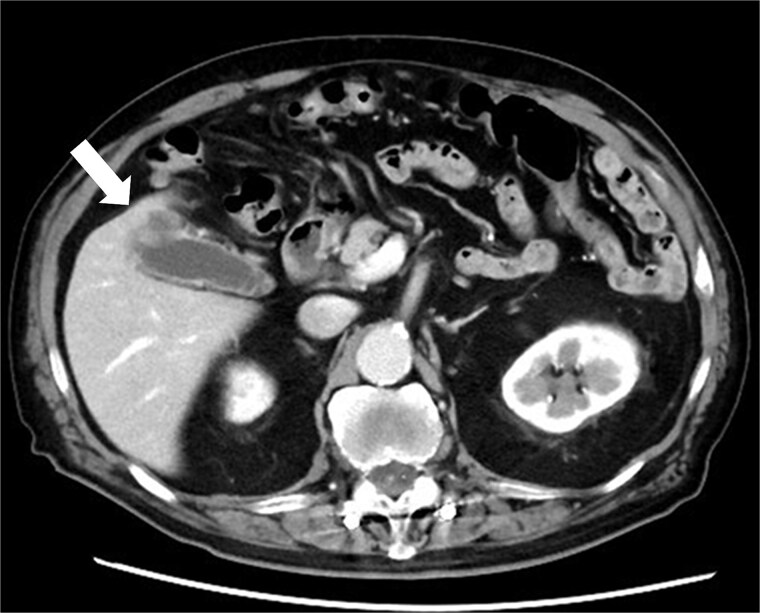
Contrast-enhanced CT showing an irregularly enhanced mass in the gallbladder fundus with suspected partial liver invasion (arrow).
